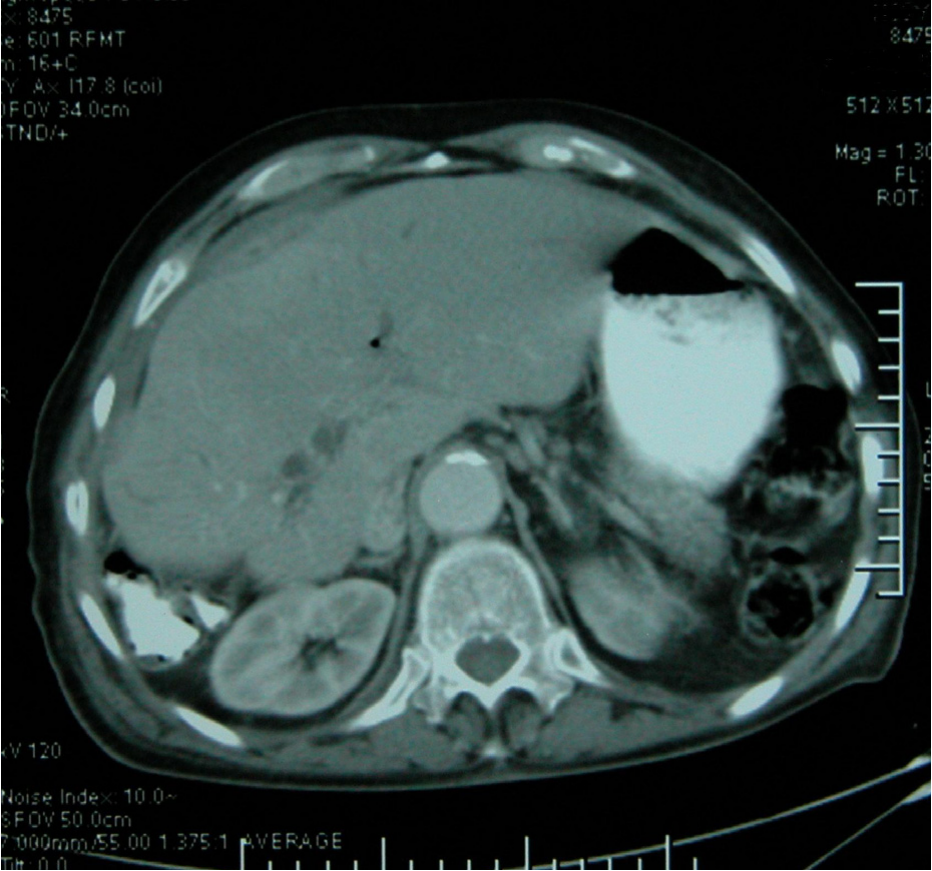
Abdominal CT showing complete resolution of the biloma after management.
